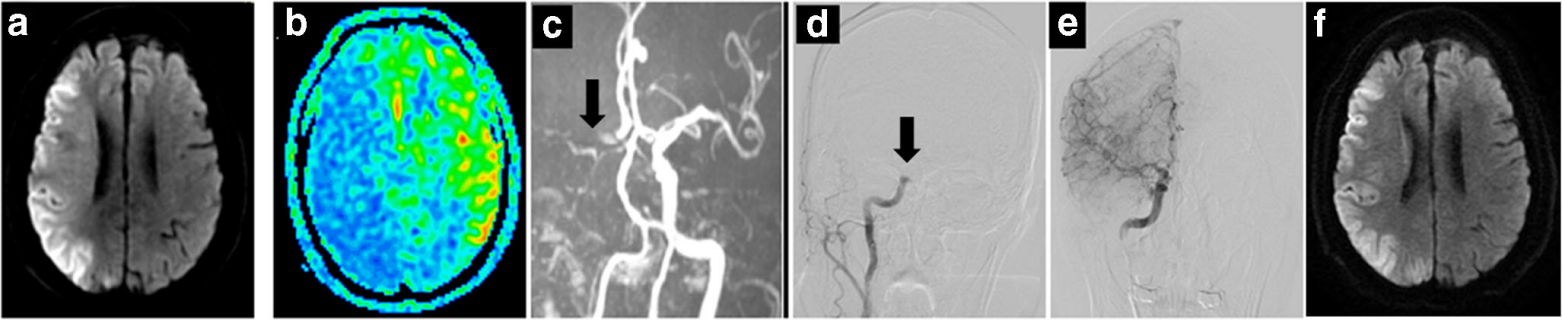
A 85-year-old man (NO. 3 in Supplementary Tables 1 and 2) with a DWI-ASPECTS of 2 and an ASL-DWI mismatch ratio of 1.95, received bridging therapy and obtained successful reperfusion at 155 min from onset. This patient achieved a favorable outcome as 3 months (mRS = 2). a Infarction lesion presented on DWI (shown as high signal). b Hypoperfusion area shown on ASL-CBF map (blue). c Right ICA occlusion shown on MRA (black arrow). d DSA showing occlusion site of right ICA (black arrow) before EVT. e DSA showing reperfusion of occlusion vessel after EVT. f DWI image at 24 h after EVT
